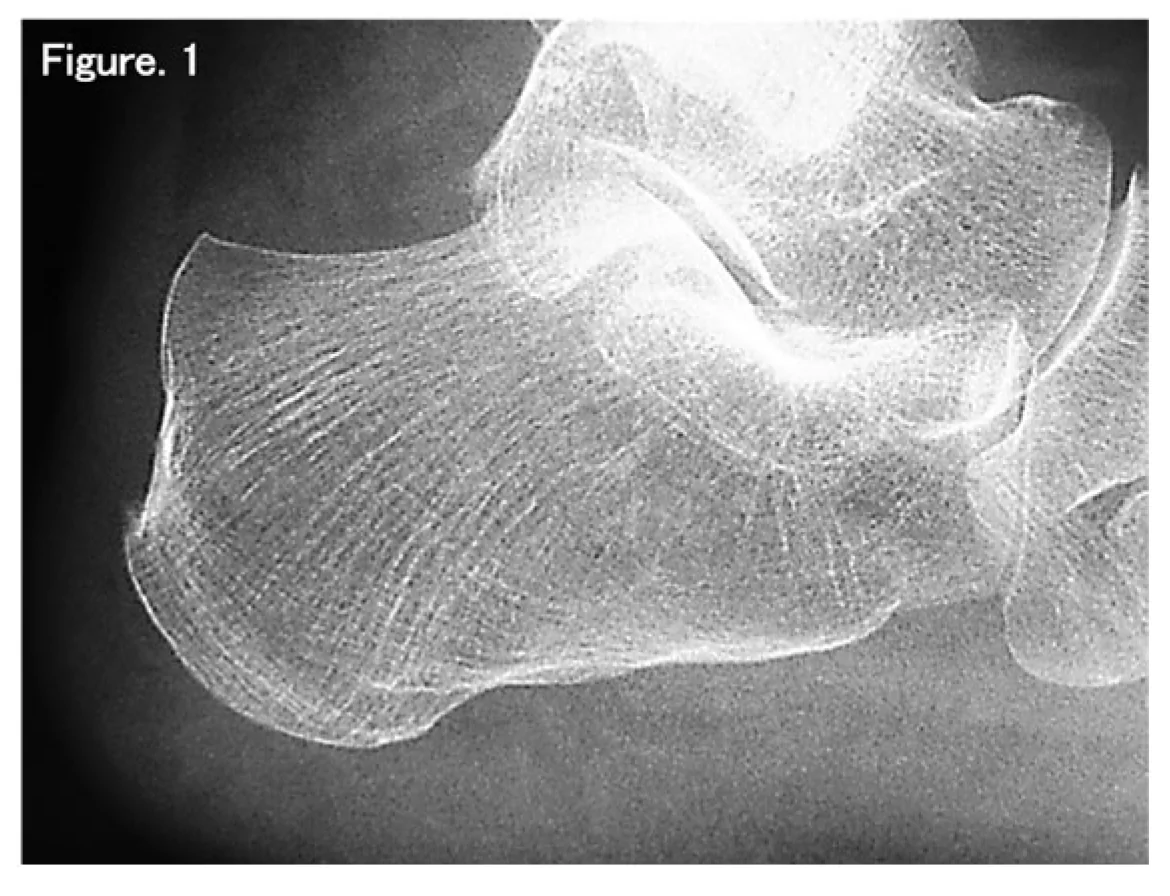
Initial radiograph of a 77-year-old woman. No definite fracture line is visible at the first visit despite atraumatic heel pain.
